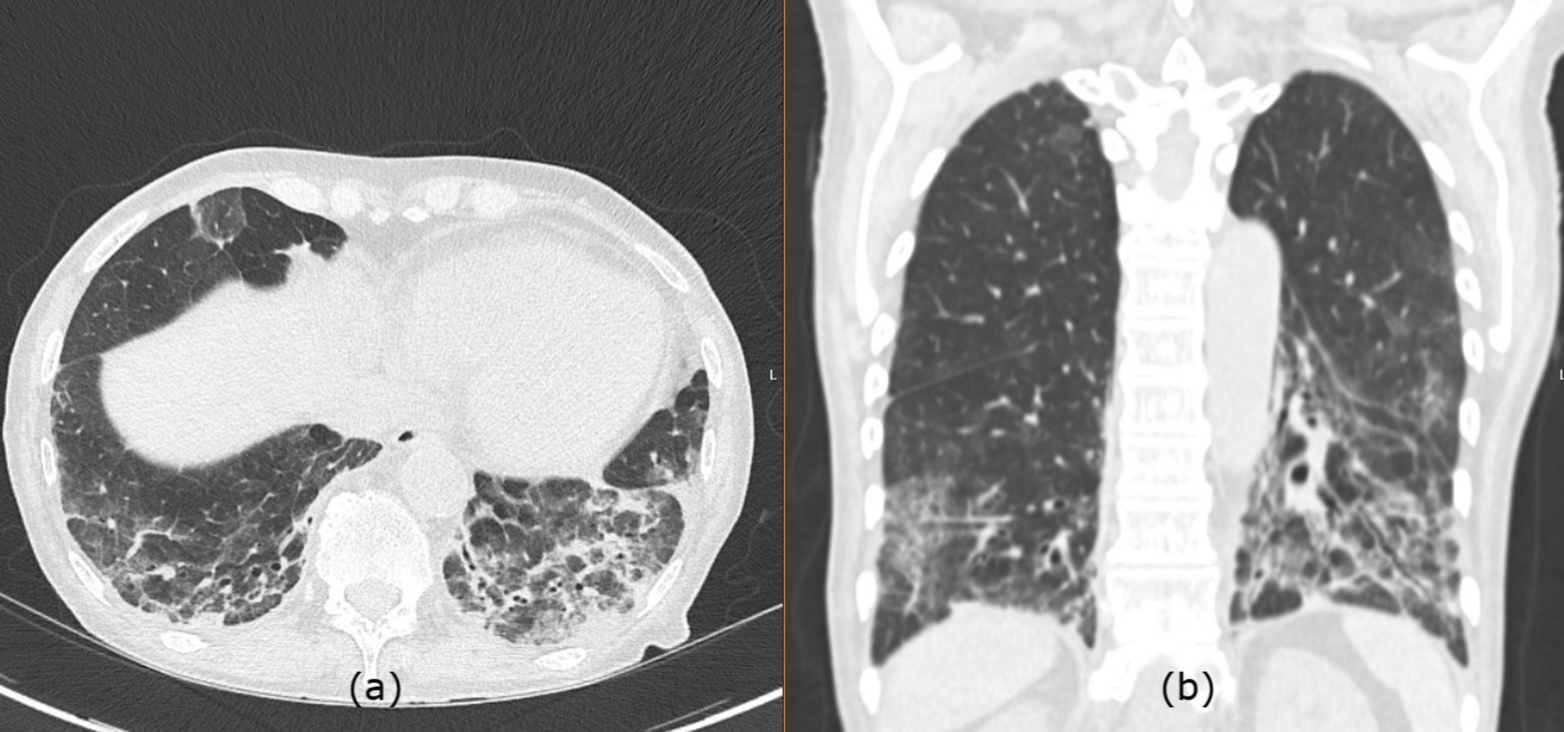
Initial CT scan after treating with obinutuzumab showing lower zone predominant peri‐broncho‐vascular consolidation with admixed ground‐glass and volume loss with tractional bronchiectasis which are evident in both axial (a) and coronal (b) images, suggesting organising pneumonia.
